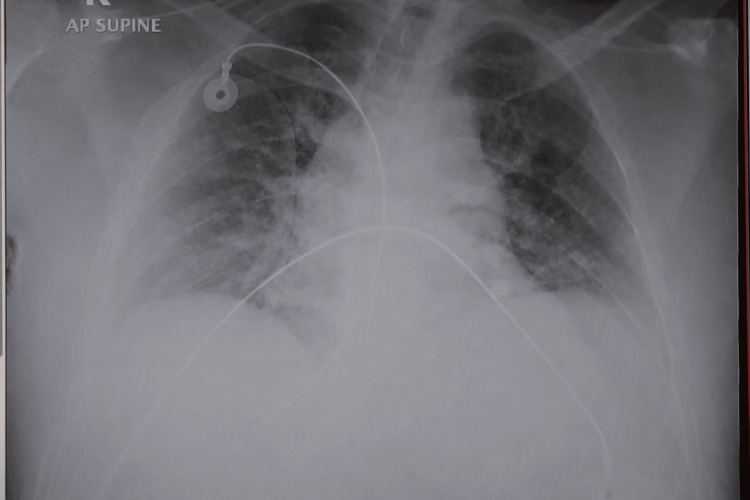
Chest X-ray of the patient on admission showing moderately bilateral congested lungs
